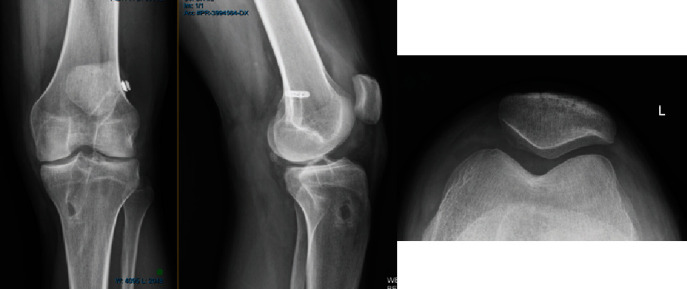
Radiograph in 2016 of BP's left knee.
